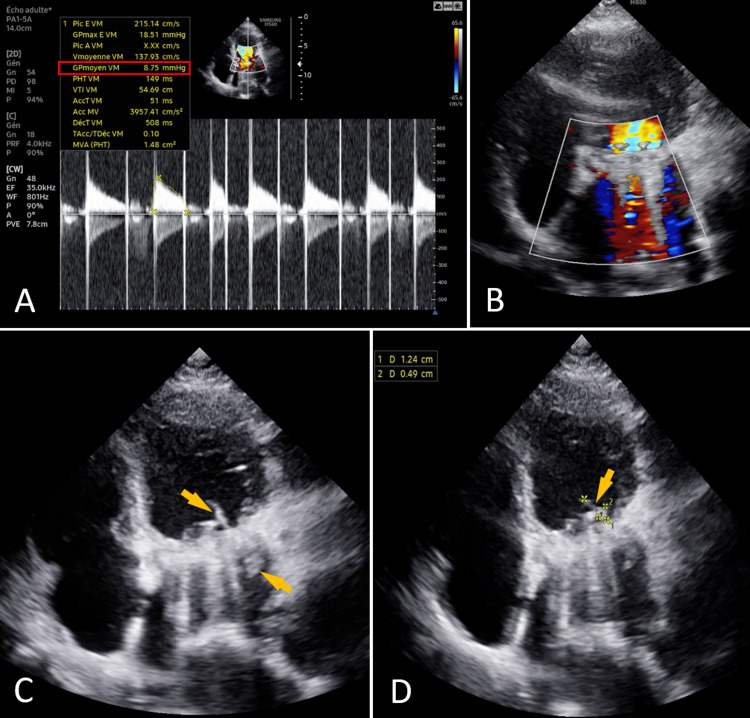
A: Elevated trans-prosthetic gradients (8.75 mmHg) on continuous Doppler, suggesting prosthetic dysfunction. B: TTE apical 4-chamber view suggesting obstruction of the mechanical mitral prosthesis on color Doppler. C and D: TTE apical four-chamber view: Vegetations on the bileaflet mechanical prosthesis in the mitral position (Arrows). TTE: Transthoracic Echocardiography
